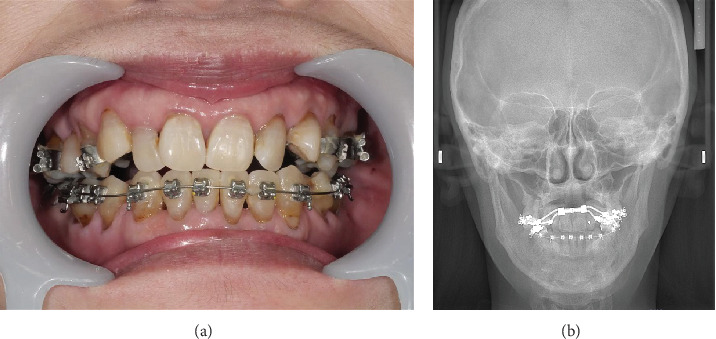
Findings from the postoperative radiograph and photograph show that the stenosis of the maxillary dental arch has improved (a and b).
